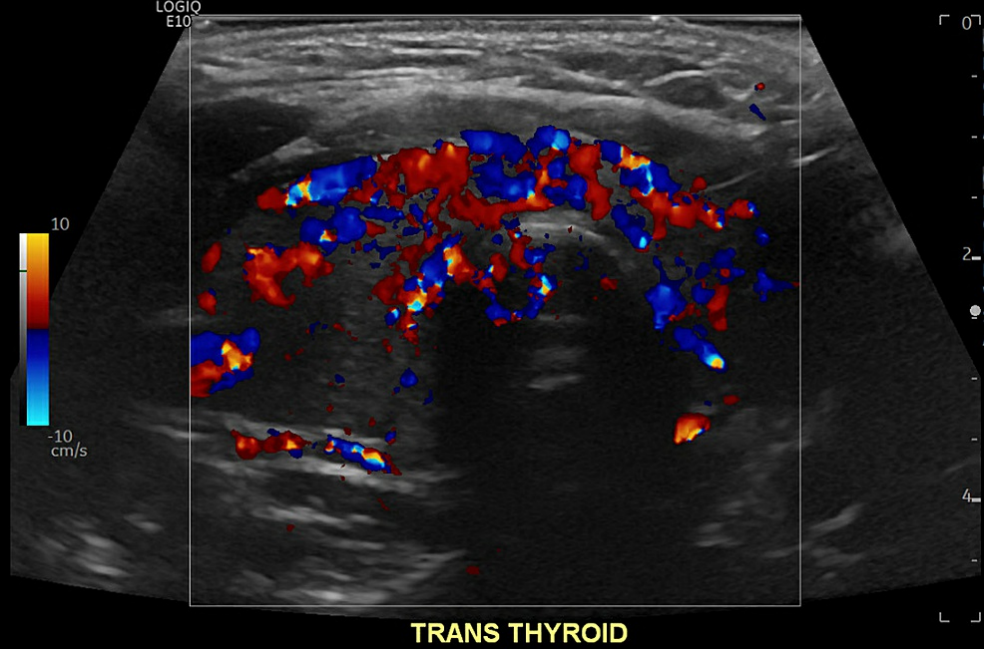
Thyroid Doppler with increased vascularity
